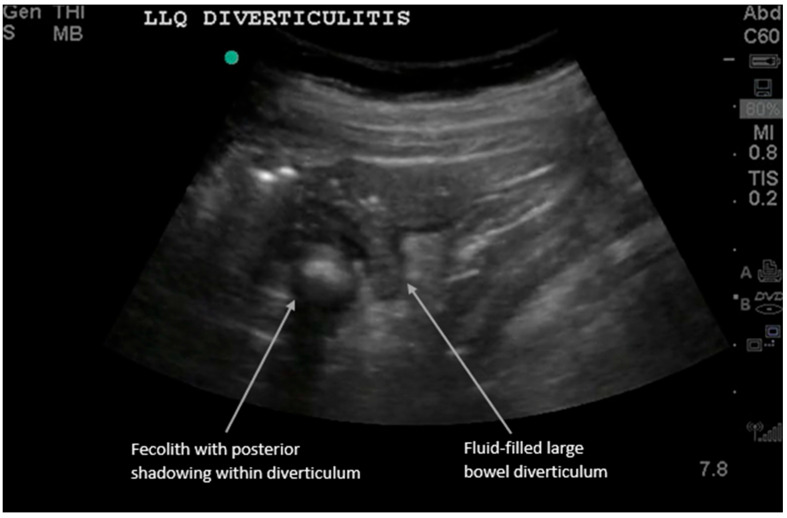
Acute diverticulitis results from inflammation of a colonic diverticulum and most commonly presents with left lower quadrant pain since it typically involves the descending or distal sigmoid colon. The sonographic diagnosis of diverticulitis, as detailed in recent comprehensive reviews, relies on visualizing diverticula as small outpouchings from the colon wall with posterior acoustic shadowing if gas or feces are present [2,5,13,14,15,16]. We present a long-axis view of large bowel diverticulitis, which has the following characteristics on ultrasound: (i) thickened large bowel wall > 5 mm, (ii) hyperemic bowel wall with color Doppler flow mode, (iii) fluid-filled contents in the diverticula, and (iv) non-compressible hyperechoic fat around the bowel. (See Supplementary Video S1 for examples of dynamic findings.) Sometimes the diverticulum can be visualized with a fecolith, and when this obstructs the diverticular neck, it can develop into diverticulitis. Most cases are uncomplicated (85%) and improve with nonoperative antibiotic treatment. However, complicated cases can show adjacent abscesses, fistulas, obstructions, or free air [6,11,12,13,14,15,16,17].
